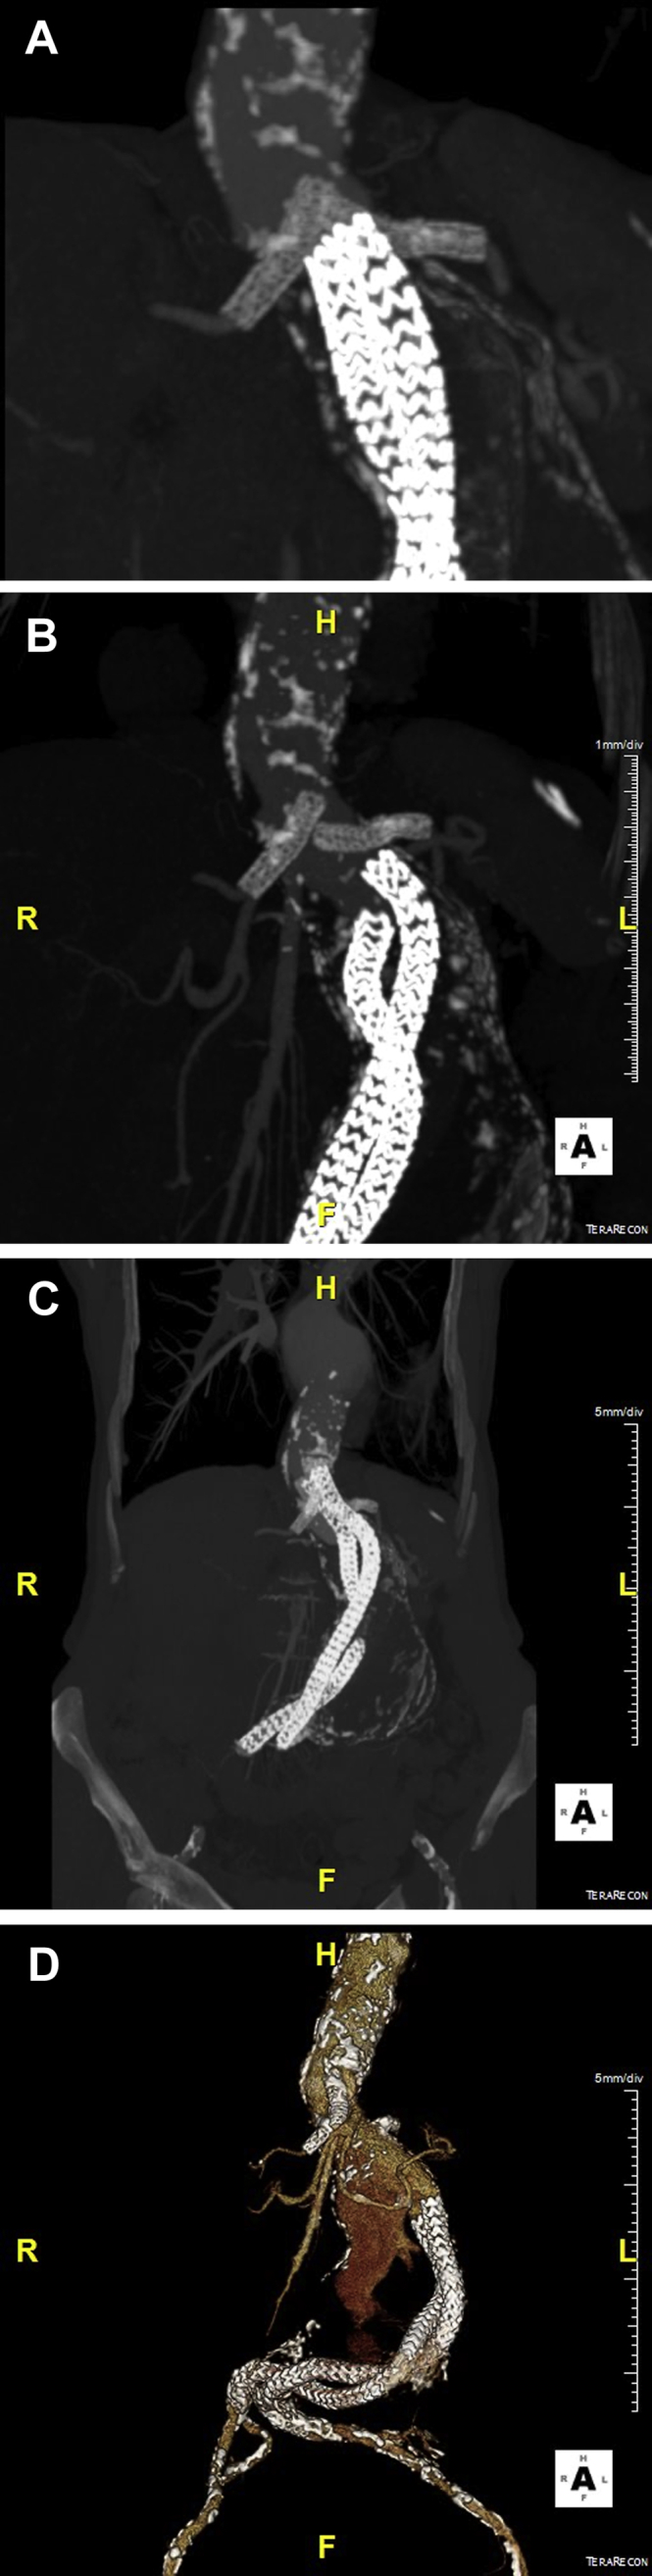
However, in November 2015, regular follow-up CTA (*B*) revealed significant migration of the Nellix stents. Open conversion was not regarded as a feasible option because of the condition of the patient. Therefore, a Nellix-in-Nellix extension including proximal extension of both renal chimney grafts and additional placement of a chimney into the superior mesenteric artery was performed. Again, the intervention was successful, and postoperative CTA demonstrated adequate placement without complications (*C*).
